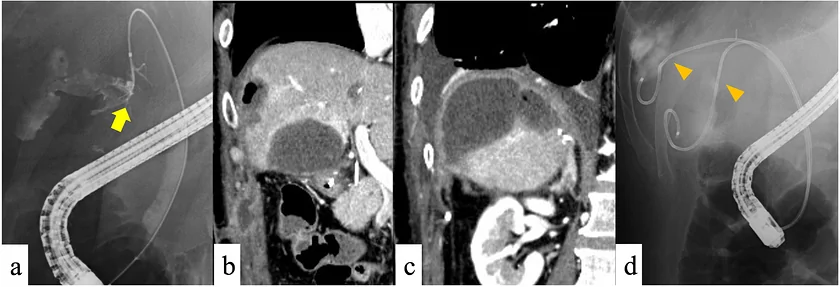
Initial endoscopic retrograde cholangiopancreatography (ERCP) findings and biloma enlargement after endoscopic nasobiliary drainage (ENBD) placement, and transpapillary/transfistular (TP/TF) drainage. (a) Fluoroscopic image during initial ERCP on postoperative day (POD) 6: the arrow indicates high‐grade bile leakage from B6, visible before complete intrahepatic opacification. (b) Coronal computed tomography (CT) on POD 11: an enlarged biloma (93 mm) in hepatic segment 6 (B6) after ENBD placement. (c) CT on POD 11: an enlarged biloma (71 mm) in segment 7 (B7). (d) Fluoroscopic image during TP/TF drainage on POD 14: two 5‐Fr pigtail‐type nasobiliary drainage tubes were advanced through the leak sites (arrowheads) and placed within the respective biloma cavities.
